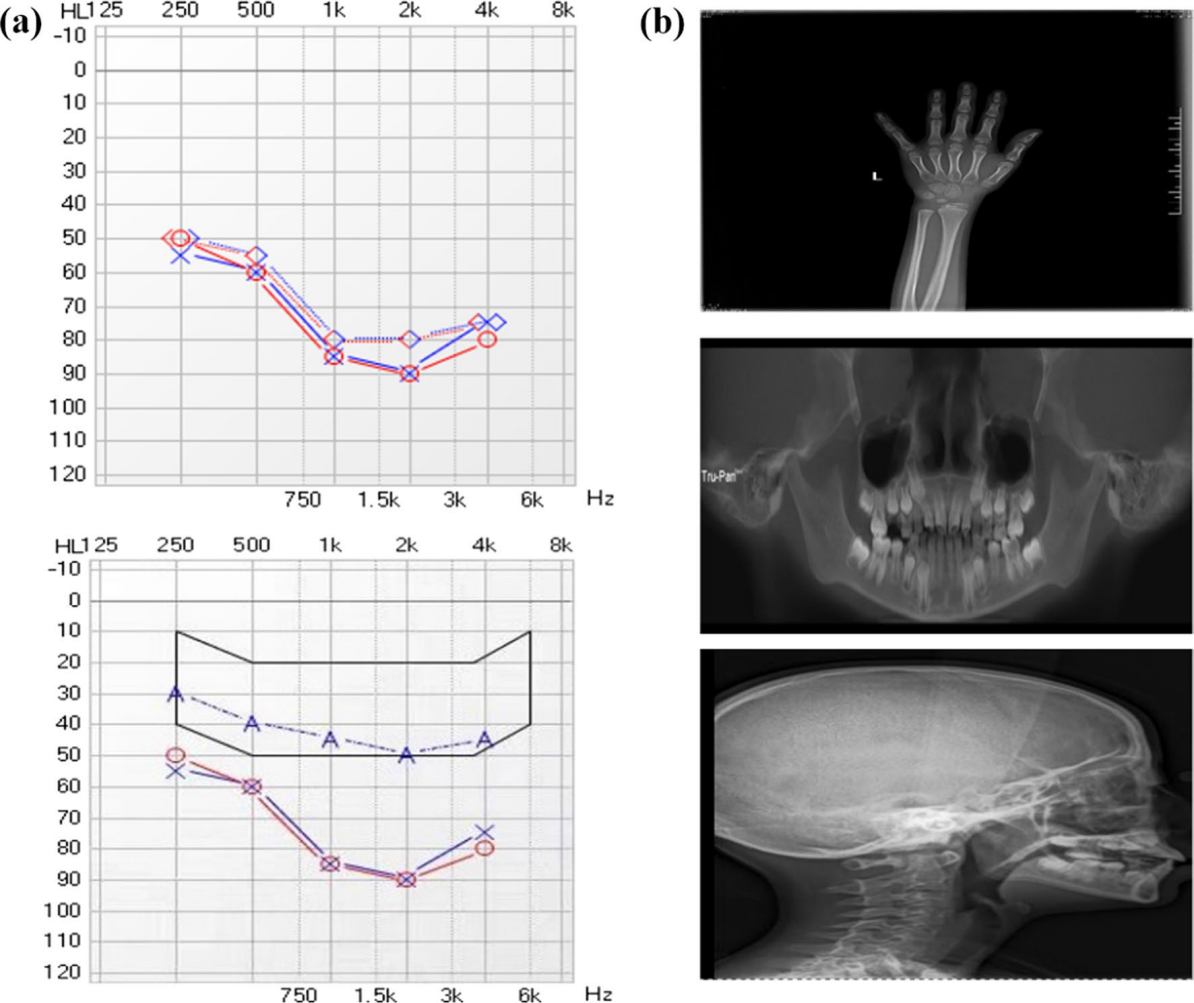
Facial phenotype, audiogram, and X-ray of the proband. a Bilateral severe sensorineural hearing loss revealed by pure-tone audiometry showed. The use of hearing aids led to a successful outcome. b When she was 6 years old, the bone age was only 3 years. X-ray showing crowded teeth and mandibular hypoplasia
